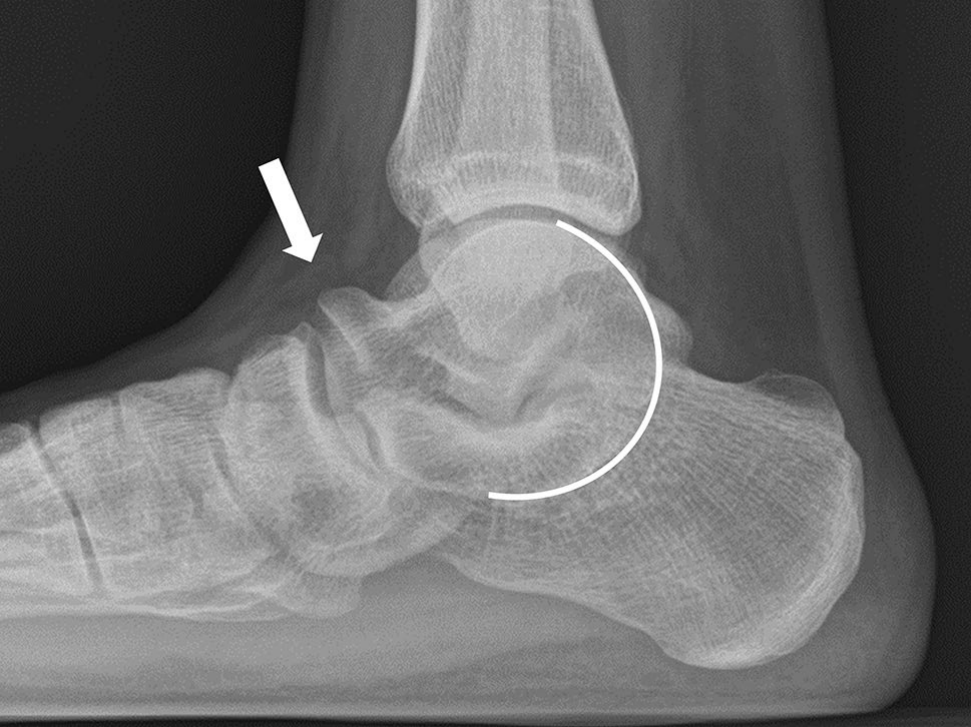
Talar beak sign and C sign on a simple radiograph.
